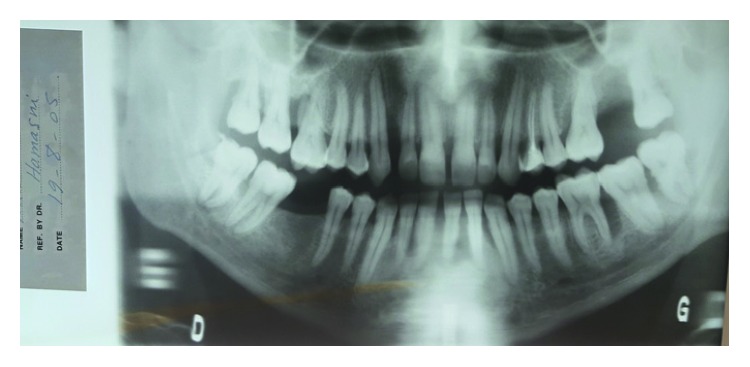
Preoperative panoramic X-ray showing generalized bone loss and missing teeth 27 and 46.
